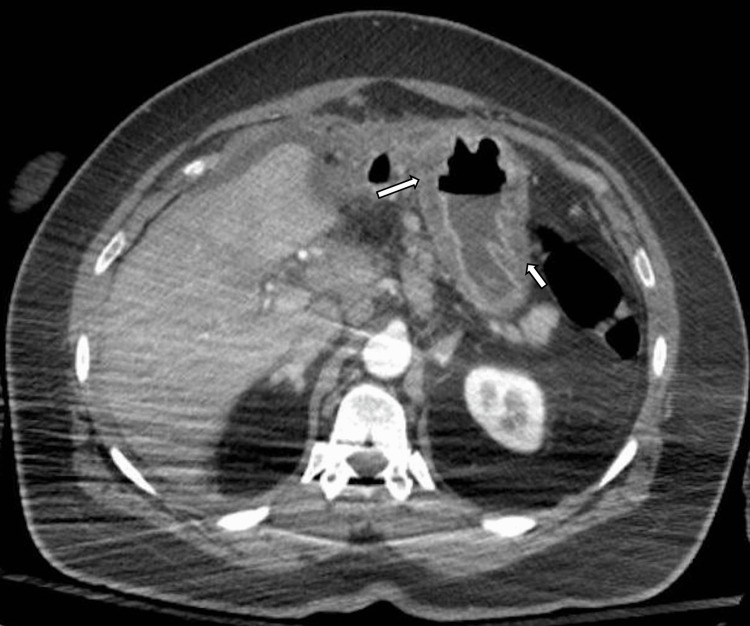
CT chest/abdomen/pelvis with contrast. CT chest/abdomen/pelvis with contrast demonstrating gastric wall thickening (arrows), perigastric stranding, edema, and free fluid.
